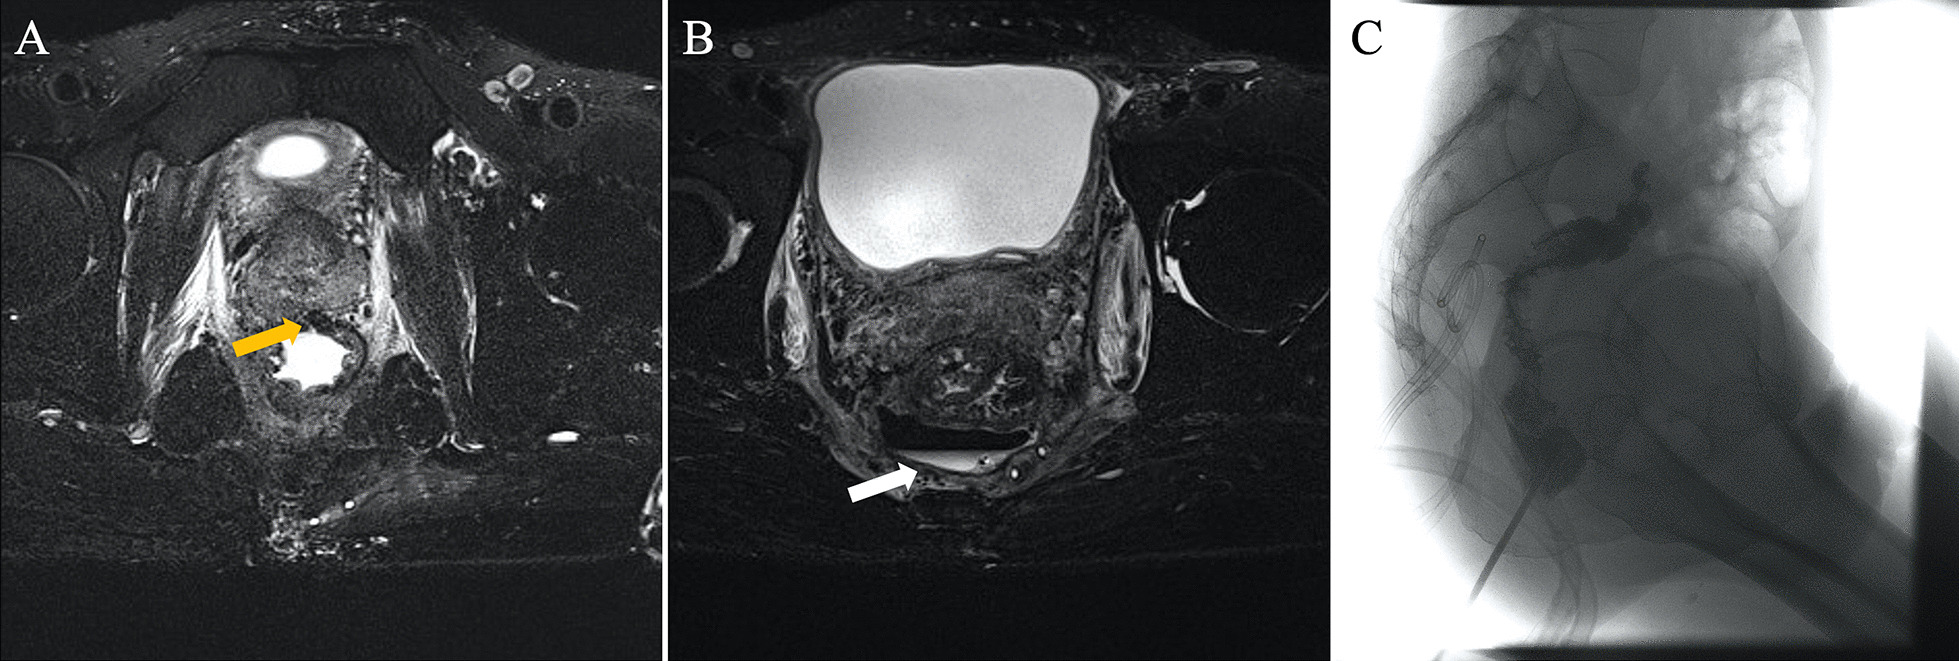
A suspected fistula tract (yellow arrow) at 12 o'clock can be seen on axial (fat suppression) T2-weighted MR images (A), suggesting anastomotic leakage in a 52-year-oldman; At a higher level image shows an abscess (white arrow) with a gas–liquid plane located in the presacral region (B). The above two signs were not found in barium enema (C)
